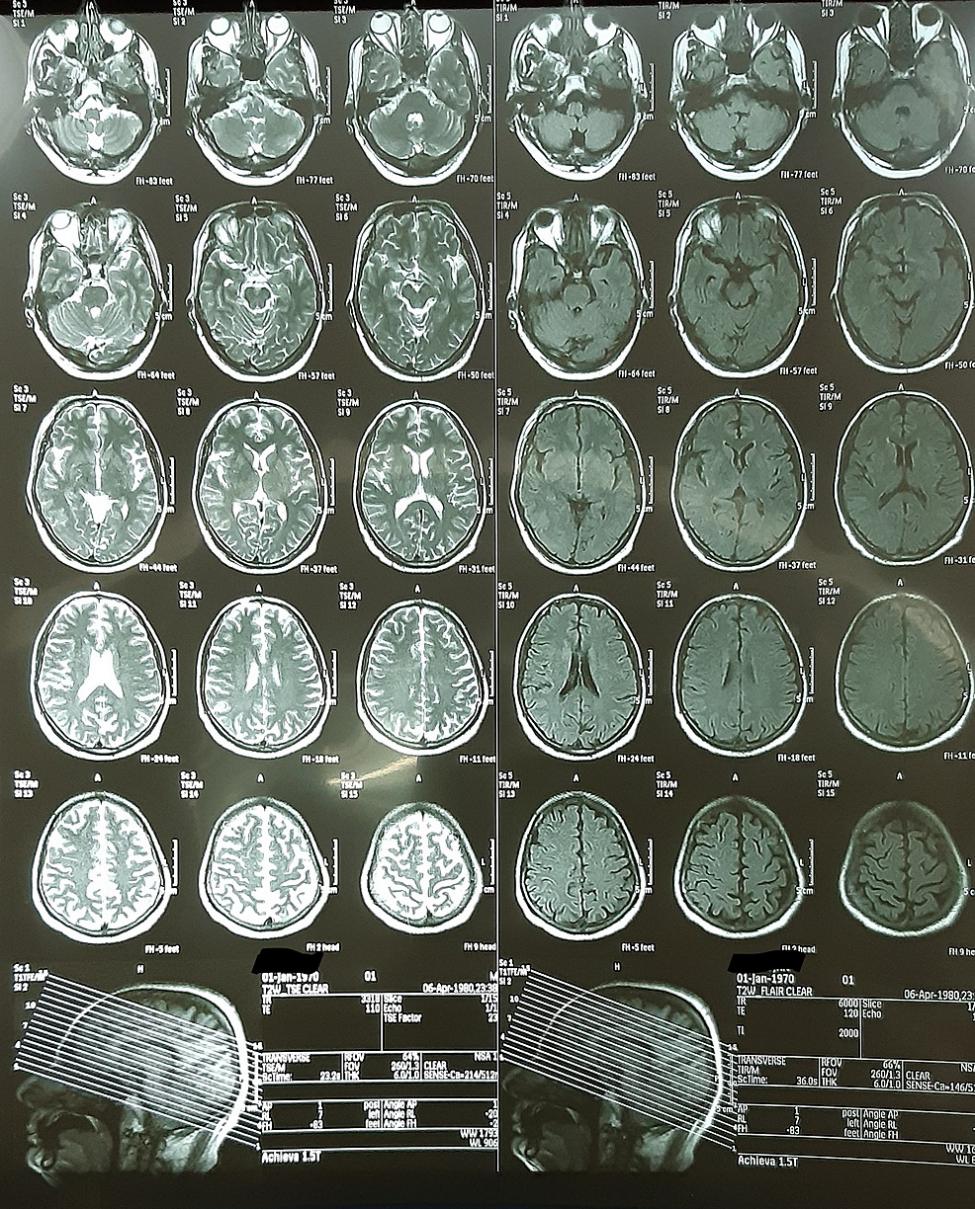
Showing transverse sections of a T2 weighted MRI- No abnormal findings present.
